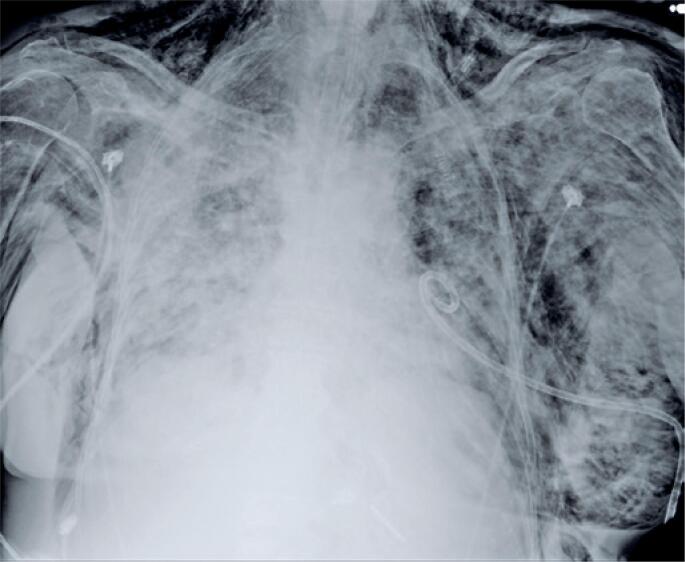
Plain chest radiograph of patient with COVID-19 and pleural fistula, with extensive thoracic and cervical subcutaneous emphysema. Presence of left pneumothorax slide and pigtail type Wayne chest drain
